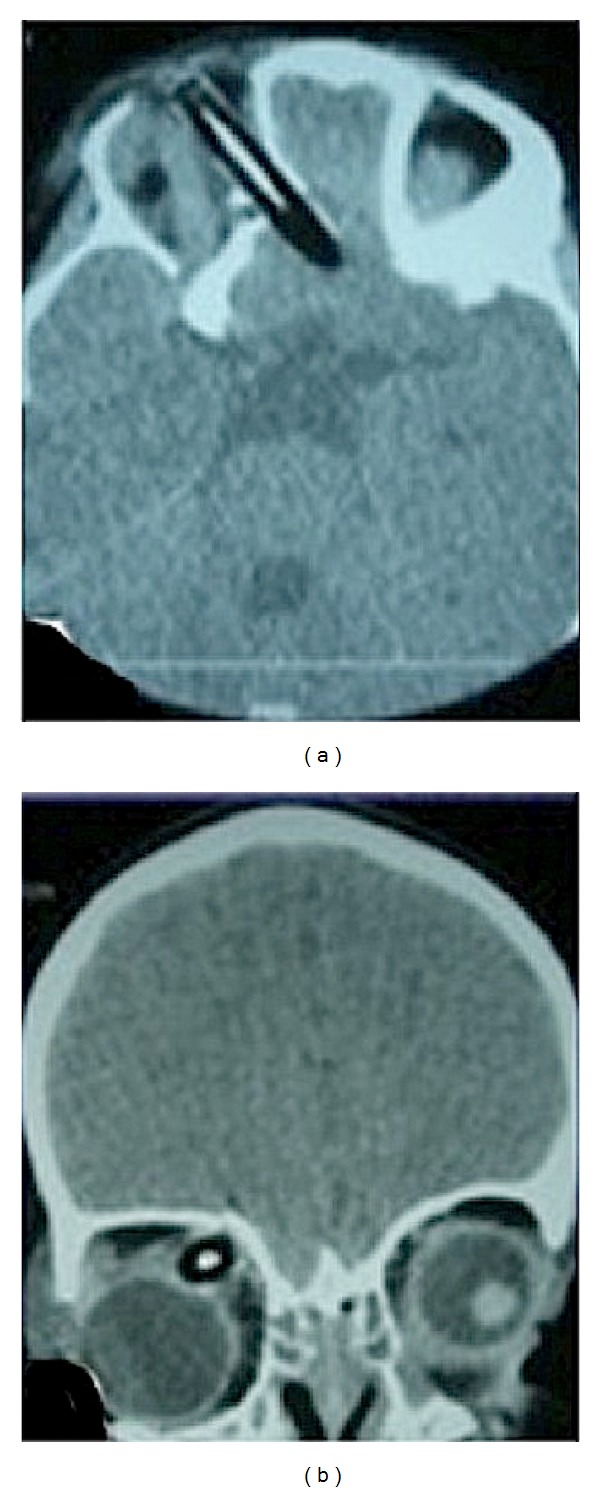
Computed tomography (CT) cranial scans demonstrating the foreign body penetration of the orbital wall (a) and downward displacement of the globe (b).
